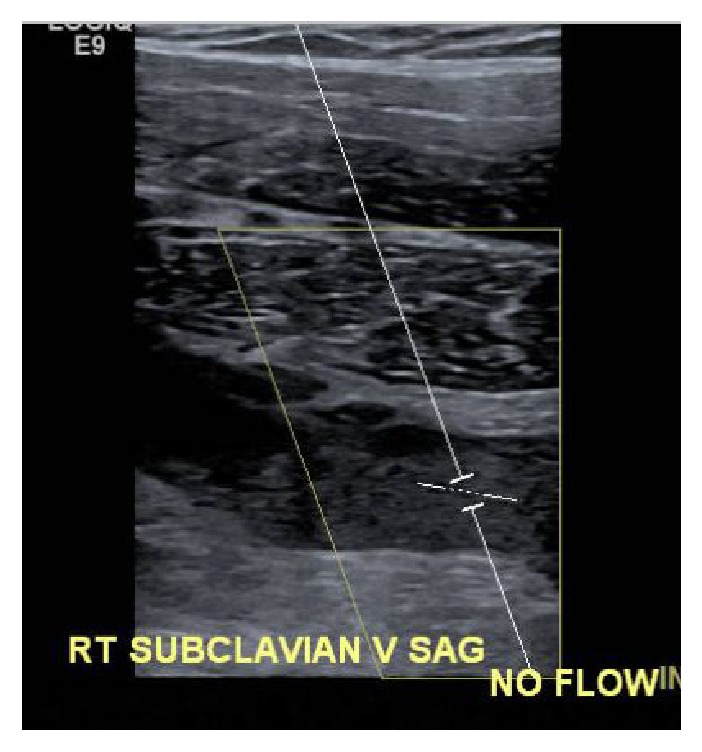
Doppler ultrasound showing absence of blood flow in the subclavian vein.
